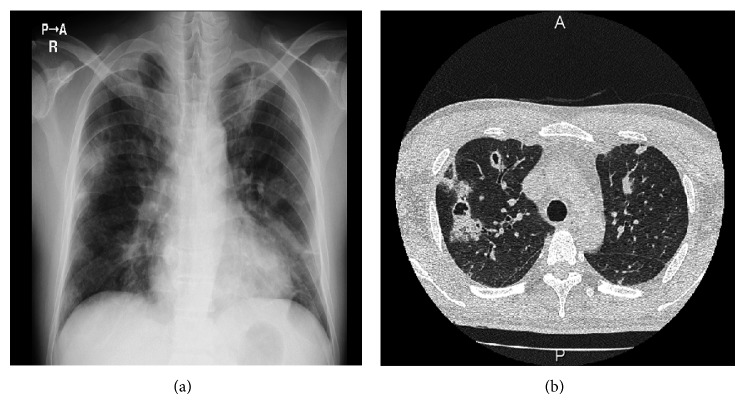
(a) Chest X-ray showing multiple bilateral nodular densities. (b) CT of the chest demonstrating bilateral multiple lung nodules, some of which are cavitated.
